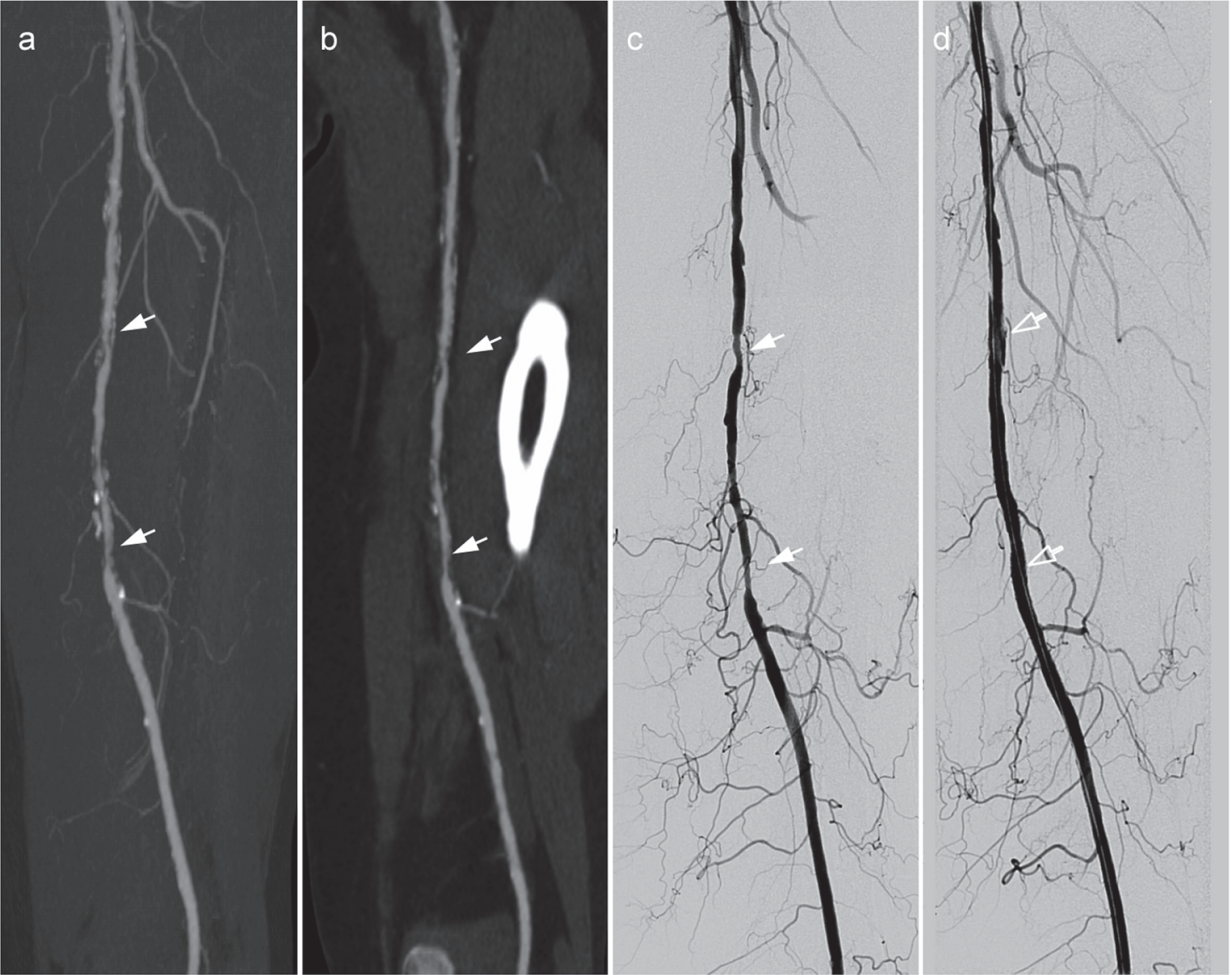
Example of a run-off CTA with sufficient diagnostic confidence and diagnostic image quality. 69 y old female with intermittent claudication of the left lower leg (Fontaine stage IIB). Run-off CTA showed multiple stenoses (white arrows) of the left superficial femoral artery (TASC B) in the MIP images (a) and curved MPR (b). Stenoses were comfirmed by DSA (c) and successfully treated by percutaneous transluminal angioplasty and stenting (d, empty white arrow).
